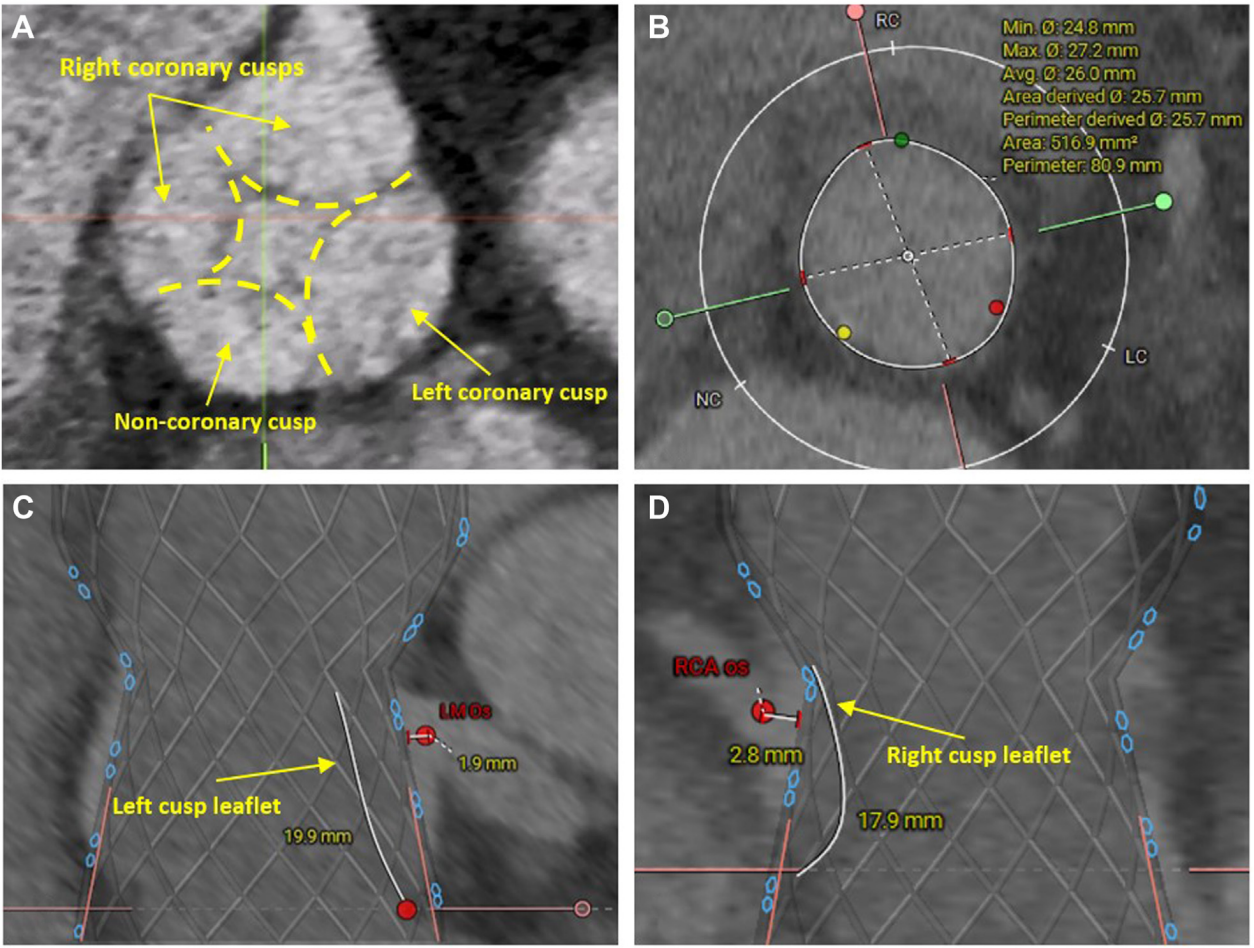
Computed tomography planning images. (A) Quadricuspid aortic valve morphology seen on cardiac computed tomography images. (B) Annular dimensions calculated during mid systole. (C,D) Predicted deployment of 34-mm Evolut FX (Medtronic) valve raising concern for coronary ostia obstruction and sinus sequestration without leaflet modification due to long leaflets (19.9 and 17.9 mm left and right, respectively) with cusps nearing the sinotubular junction and a low valve to coronary dimension and low valve to sinotubular junction dimension for both the right coronary artery and left main coronary artery.
